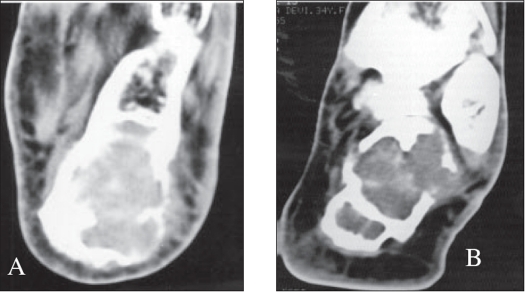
CT scan of the same lesion A) shows expansion of calcaneum. B) Shows cortical breach
